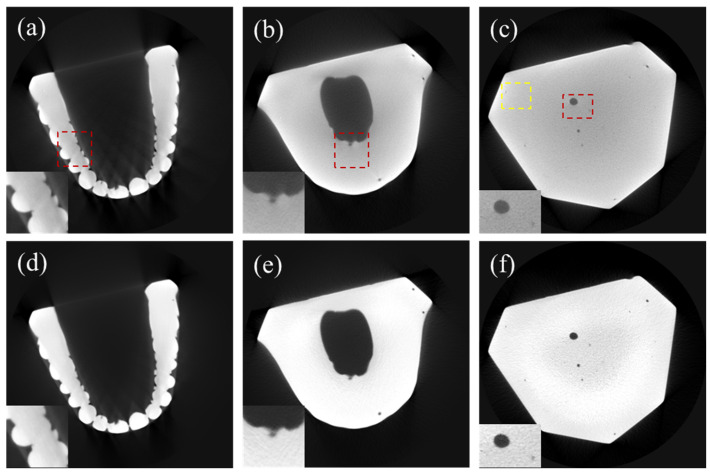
CT images of Model 1. (a–c) Uncorrected CT images. (d–f) DSC-SAC-corrected CT images. The zoomed images in the bottom left corner are related to the red ROIs while the yellow Roi is used to evaluate the signal to noise ratio (SNR).
